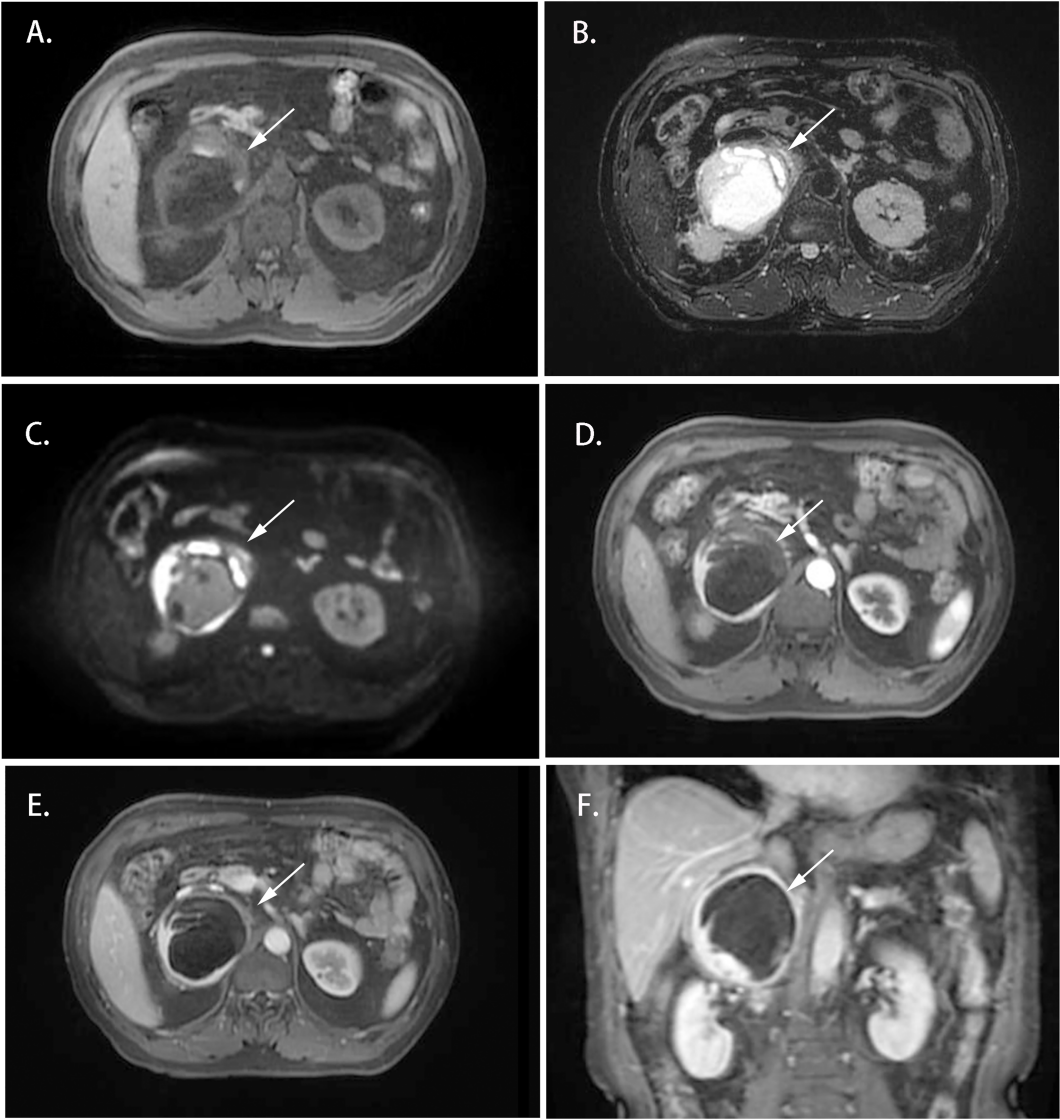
Magnetic resonance imaging of the Adrenal CD of Case 1 (white arrow). (A) Axial T1WI; (B) Axial T2WI; (C) DWI; (D) Enhanced T1WI (cortex phase); (E) Enhanced T1WI (delayed phase); (F) Coronal Enhanced T1WI. MRI scan and dynamic enhancement showed an 87 × 79 × 85 mm mass with partial hyper-intensity on T2WI and mild to moderate heterogeneous enhancement at the edge and septum of the lesion in the right adrenal region.
